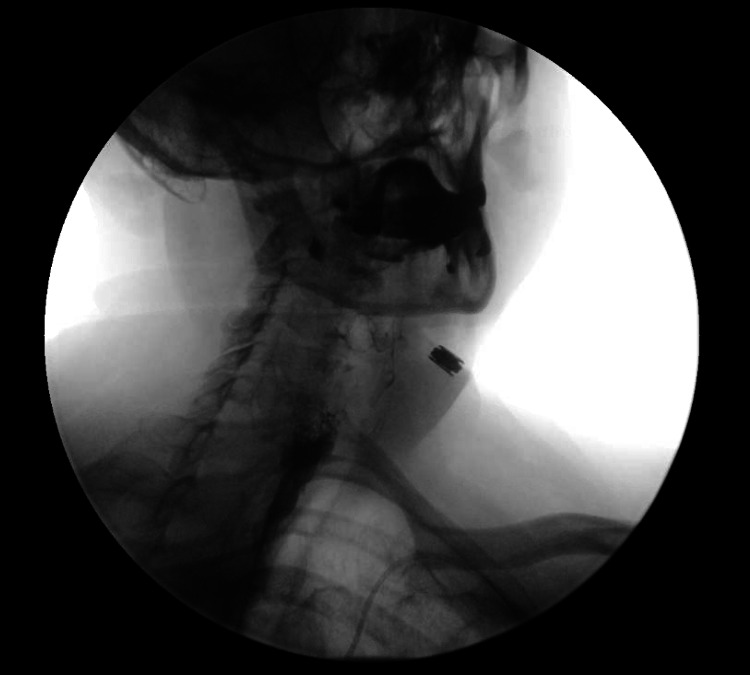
Barium swallow X-ray image taken at the end of the patient's hospital stay. Image shows no evidence of contrast leak from the esophagus, indicating that the prior esophageal perforation had healed.
